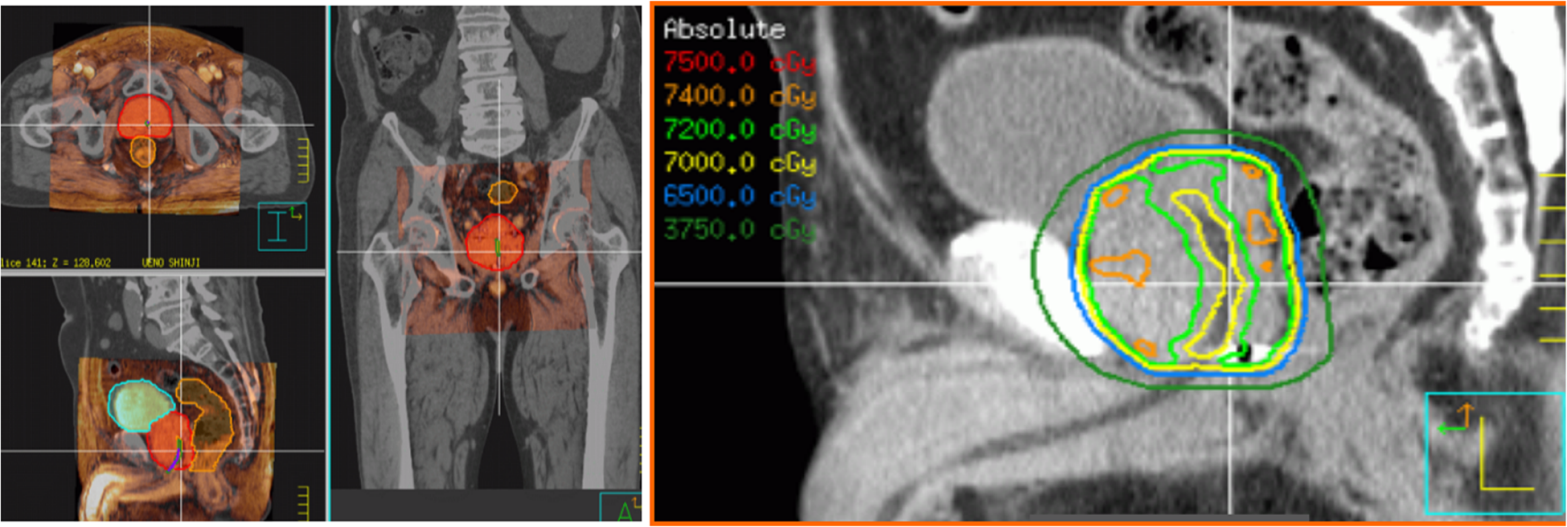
MRI image fusion for treatment planning and dose distribution for urethra dose controlled IMRT planning. MRI image fusion is used for treatment planning (left image). IMRT planning is performed with the constraint that urethra dose will not exceed V70Gy > 10% generally (right image).
